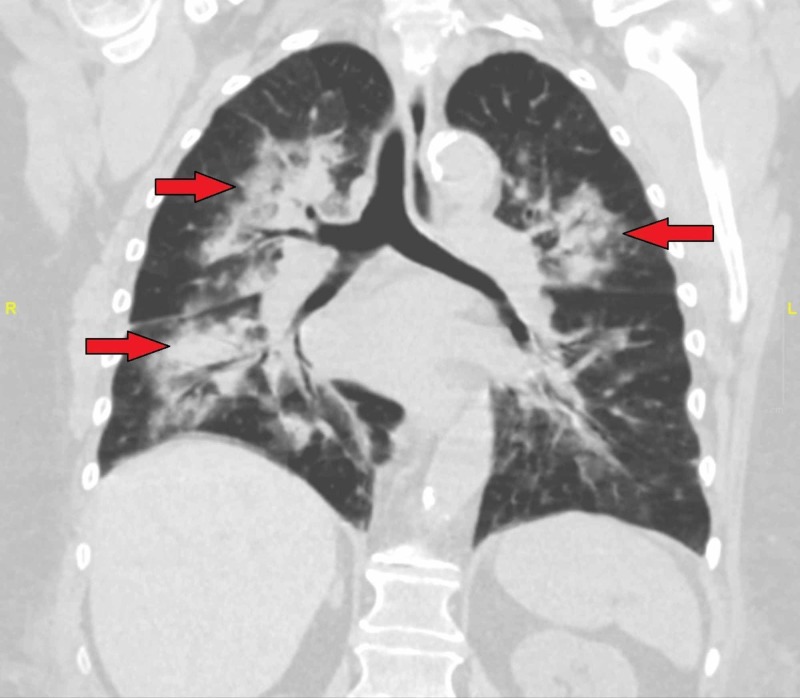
Post-hemoptysis CT of chest Red arrows indicating bilateral opacities appreciated on CT scan consistent with diffuse alveolar hemorrhage vs. pneumonia. Diffuse alveolar hemorrhage was subsequently confirmed on bronchoalveolar lavage.
